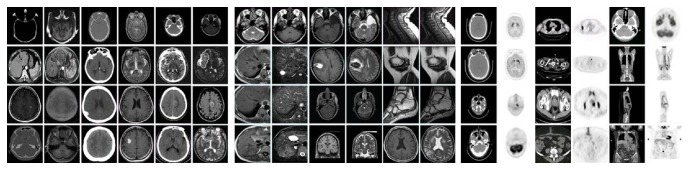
The source images for fusion and training dictionary, including 12 pairs of CT/MR images, 12 pairs of MR-T1/MR-T2 images, and 12 pairs of CT/PET images.
